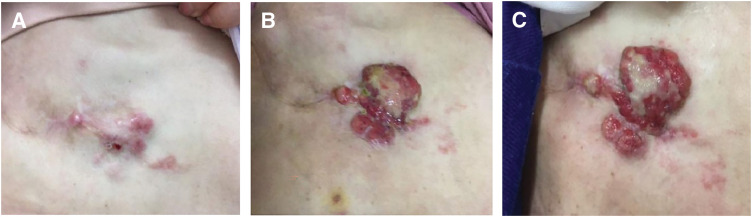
The course of chest wall recurrence lesions. (A) Chest wall recurrence at initiation of chemotherapy. (B) Treatment range for chest wall tumors defined by CT imaging. (C) Chest wall tumor condition before starting irradiation with needles placed.
